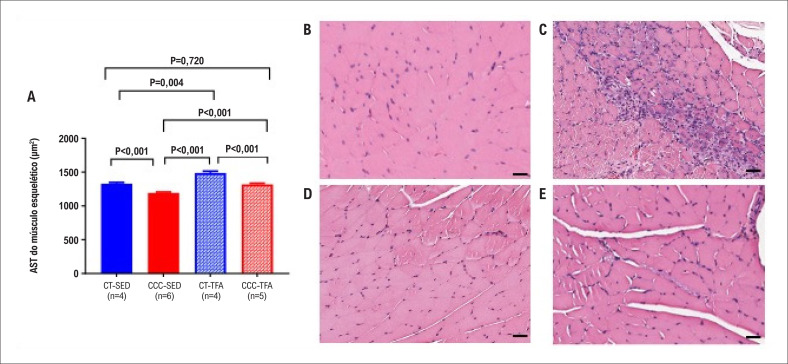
(A) Gráficos de barra mostrando a análise quantitativa da área de secção transversa do músculo gastrocnêmio de animais do grupo infectado e do grupo controle. Imagens histopatológicas representativas do músculo gastrocnêmio dos grupos (B) CT-SED (n=4), (C) CCC-SED (n=6), (D) CT-TFA (n=4) e (E) CCC-TFA (n=5). Infiltrado inflamatório difuso de células mononucleares pode ser visto em CCC-SED (C); TFA: Treinamento Físico Aeróbico; CCC: Cardiomiopatia Chagásica Crônica; CT: Controle; SED: Sedentário; escala =100 μm, aumento 20x.
